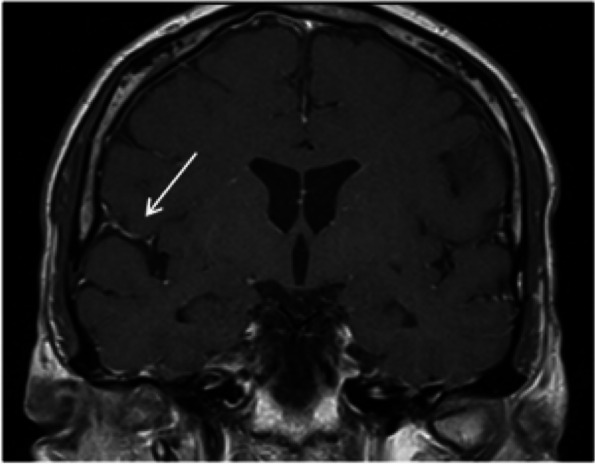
Brain MRI-T1 weighted post-gadolinium sequence showing an asymmetrical meningeal thickness with gadolinium enhancement at the right temporal lobe
